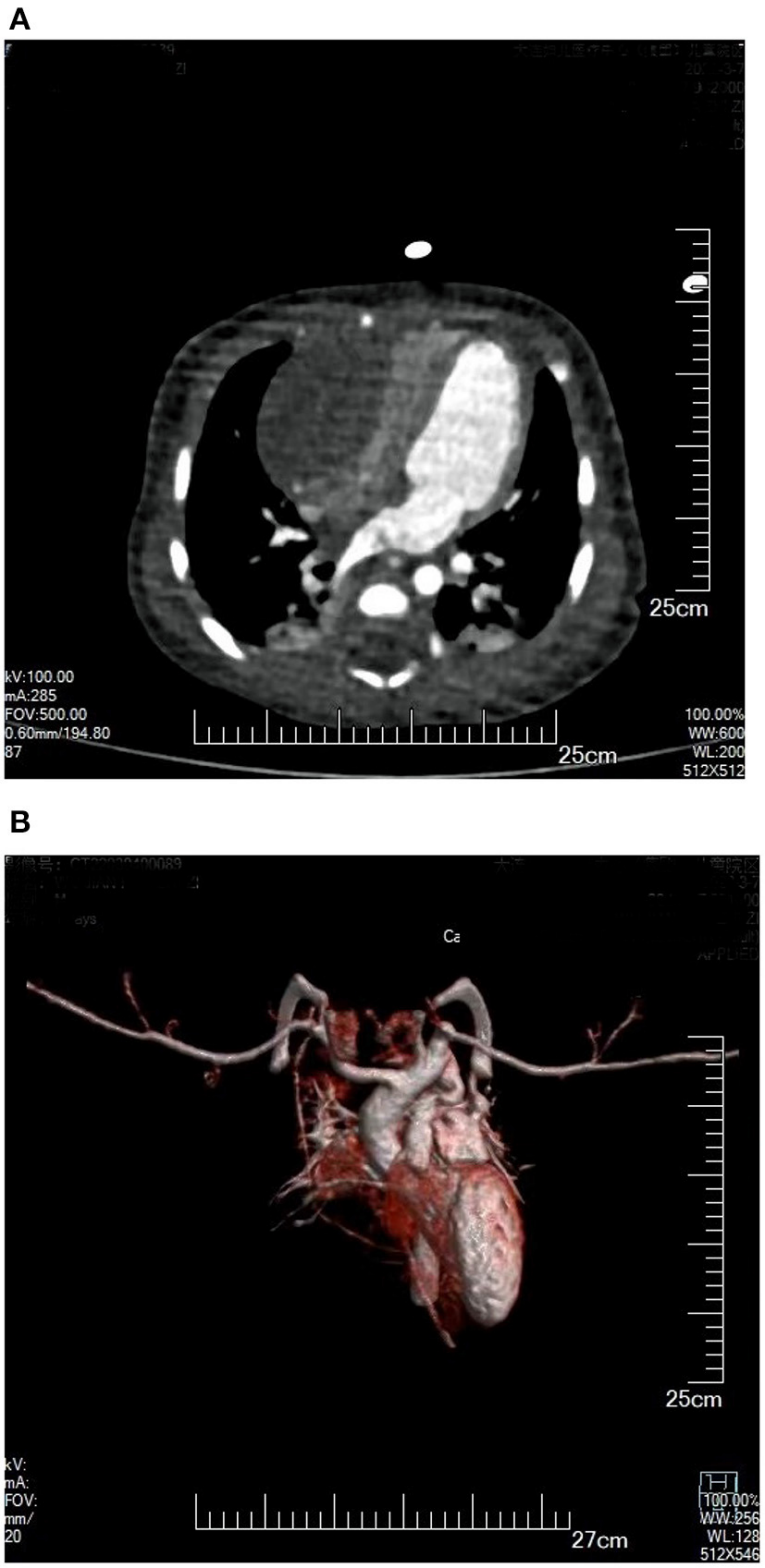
(A) Plain CT scan: Enormous space-occupying mass on a right ventricular wall, with compressed right ventricle cavity. (B) 3D reconstruction of cardiac blood flow: The right ventricular inflow tract was compressed by tumor, without obvious blood flow filling.
